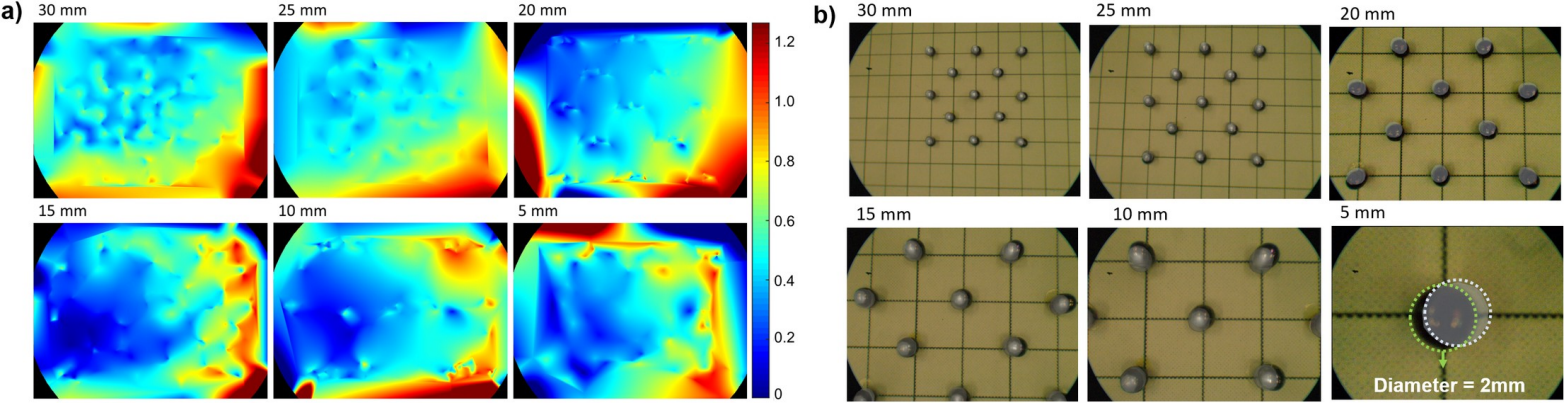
a) Error distribution, expressed in mm, of the image overlay on the endoscopic view at several distances between the endoscope and the grid. Blue represents areas with lower TRE, and red indicates areas with larger TRE. b) Steel spheres segmented from the CBCT and overlaid on the endoscopic view at several distances between the endoscope and the grid.
